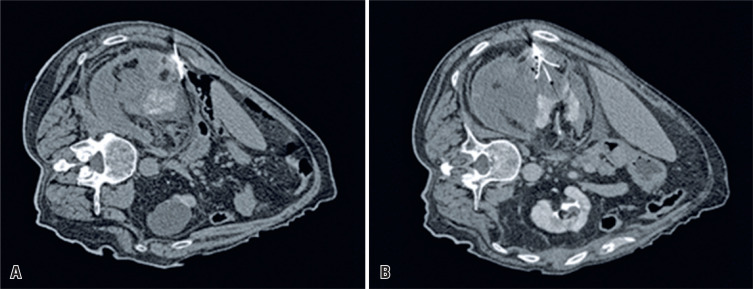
Sequential intraprocedural axial computed tomography images. (A) Hydro-dissection of the anterior surface of the right kidney with the radiofrequency ablation probe placed in the deep interface of angiomyolipomas and renal parenchyma; (B) Confirmation of the position of probe lines and radiofrequency ablation
